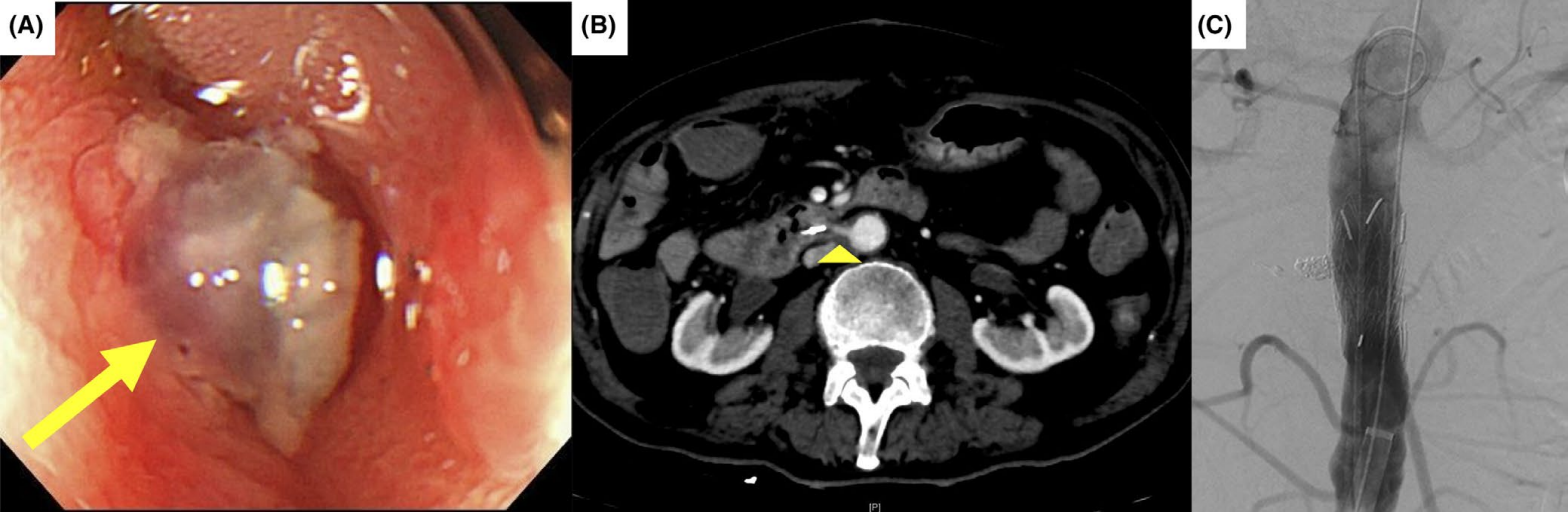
A, Gastrointestinal endoscopy of the duodenum. The endoscopy revealed a duodenal ulcer with a pulsating vasculature (arrow). B, Contrast‐enhanced computed tomography arterial phase. The arterial phase showed the aorta directly communicating with the duodenum (arrowhead). C, Aortography after endovascular aortic repair. Excluder aortic cuff (2.3‐3.3 cm) was used as endovascular aortic graft.
